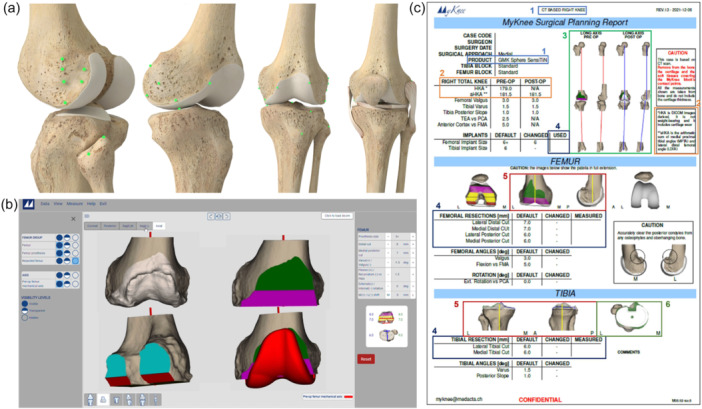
Preoperative planning. Preoperative planning using CT‐based 3D models and the MyKnee software (Medacta), including: (a) virtual 3D bone model and surface mapping; (b) resection planning for femur and tibia and (c) surgical planning report to guide kinematic alignment in total knee arthroplasty. CT, computed tomography.
